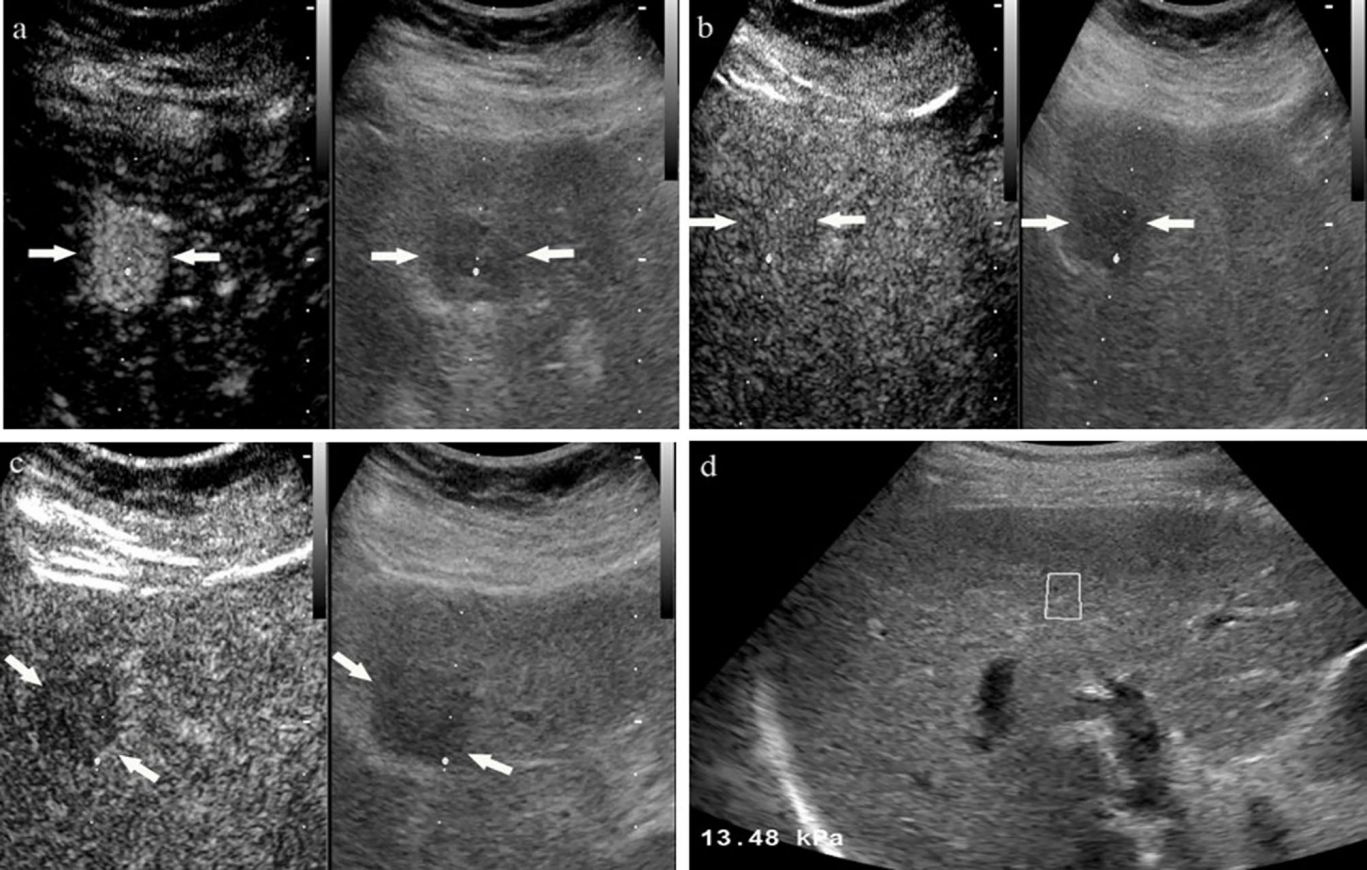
A 58-year-old male with unknown risk factors for HCC. The arrow highlights a 2.4 cm hypoechoic tumor in liver segment IV. At the arterial phase of contrast enhanced ultrasound, the mass showed rapidly whole hyper-enhancement (A), and presented as iso-enhancement at portal phase (B), finally with slightly washout at late phase (C). Ultrasound elastopraphy showed the stiffness value of liver background was 13.48 kPa (D). The lesion was proved to be moderately differentiated hepatocellular carcinoma by pathology.
